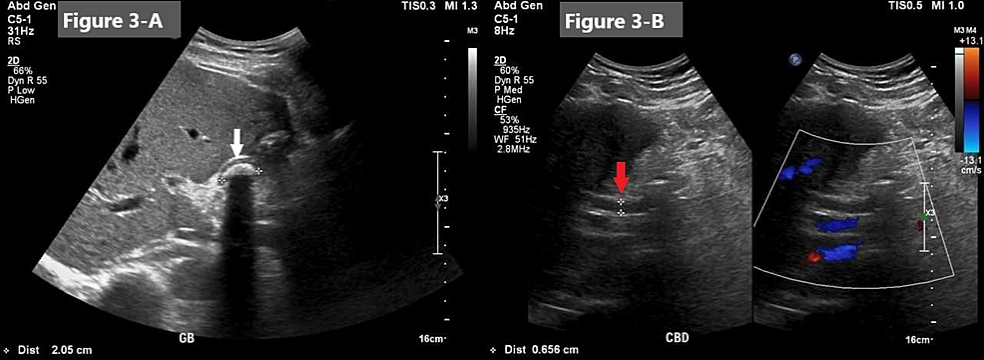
Ultrasound image (A, left): the gallbladder was contracted and demonstrated a 20 mm calculus (white arrow). (B, right): CBD was normal in caliber, measuring 5-6 mm (red arrow). CBD, common bile duct
